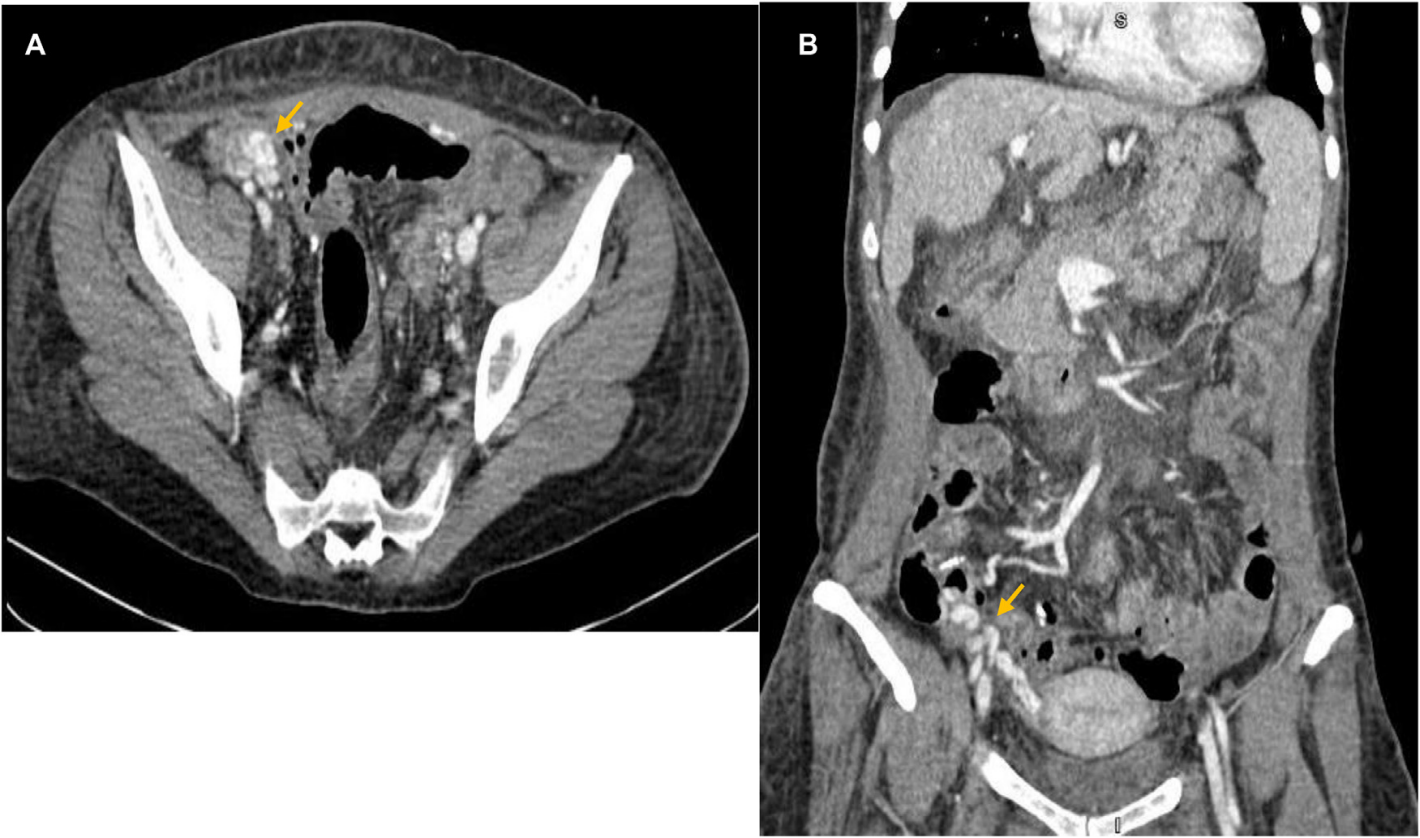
Multiphase contrast-enhanced CT images (A, B) in the venous phase (axial and coronal reconstructions) demonstrate serpiginous enhancing tubular structures (orange arrows) along the ileal loops and ileocolic territory, consistent with ectopic ileal and ileocolic varices. The largest variceal channel measures approximately 6-7 mm in diameter.
